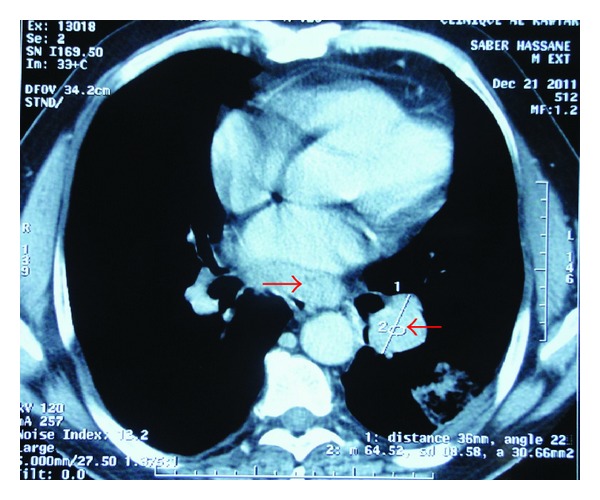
CT scan of the chest cut: extensive mediastinal lymphadenopathy with a mixed-density lung mass with irregular margins at the left hilum.
